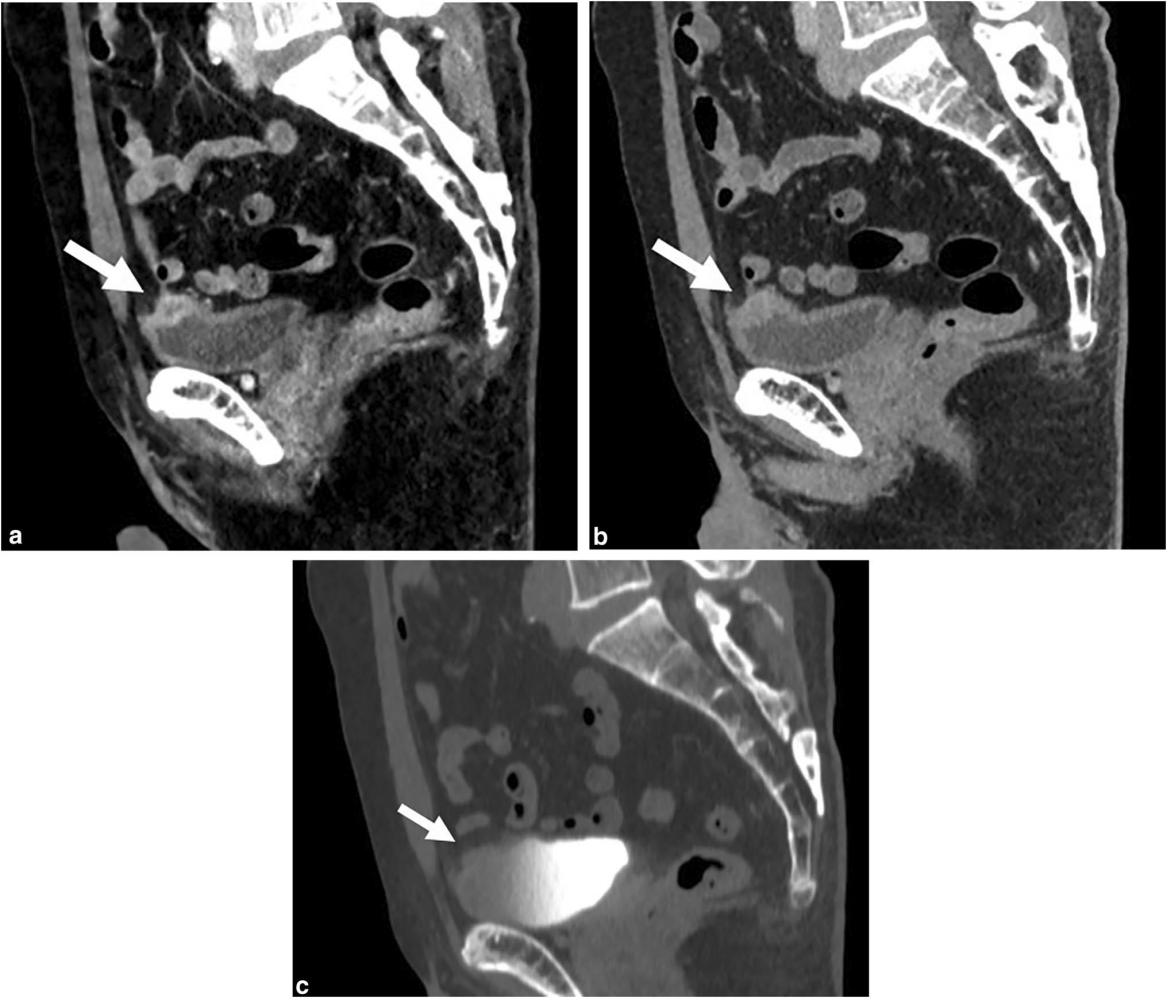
Stage pT1 invasive urothelial carcinoma with divergent differentiation in a 66-year-old man. Sagittal CT images (a 40 keV, b 120 kVp, c excretory phase 120 kVp) show a mass (arrows) on the dome of the bladder. The mass is not depicted on the excretory phase image because the anterior part of the inside of the bladder is not enhanced. The mass is more clearly depicted on 40-keV than on 120-kVp images (40 keV, grade 3; 120 kVp, grade 2; excretory phase, grade 0)
